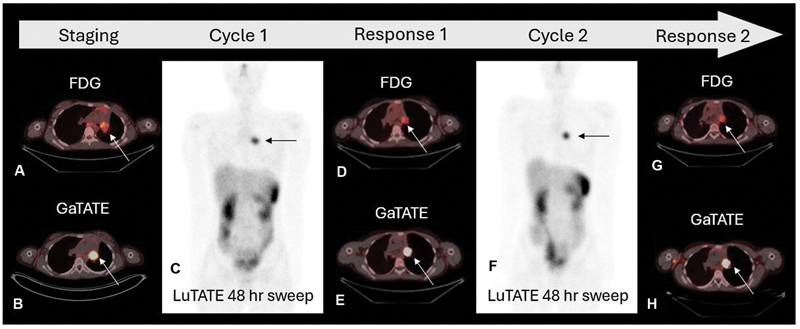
Axial fused images of the staging FDG (
